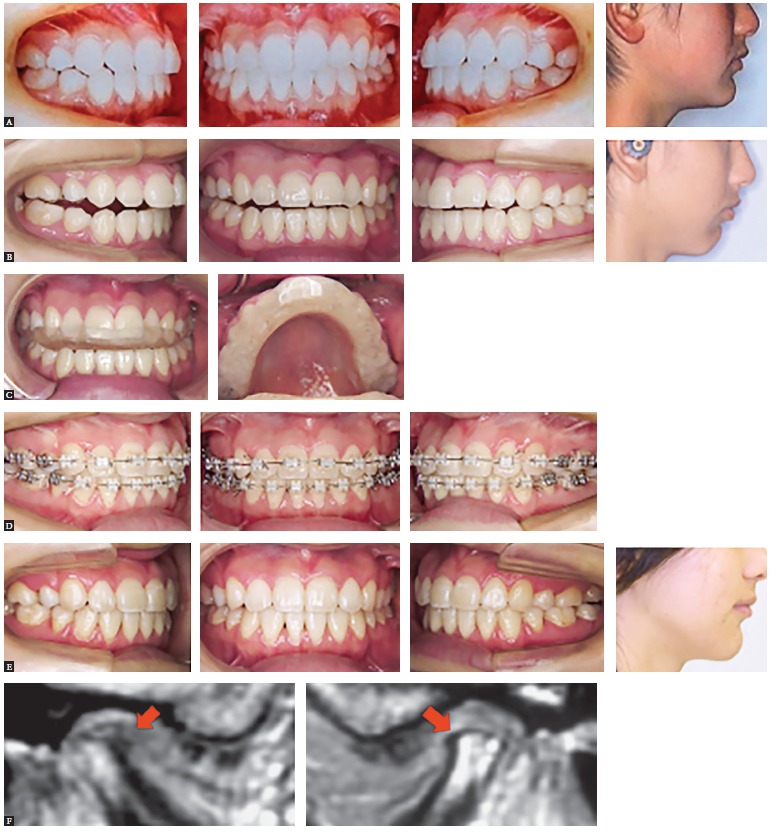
A treatment case with open bite accompanied by osteoarthritis of the temporomandibular joint. A) Initiation of retention at a previous hospital. B) Initial arrival at our hospital. C) Initiation of splint therapy. D) Orthodontic treatment with multi-bracket appliances. E) Removal of all appliances. F) MRI indicated TMJ-osteoarthritis on both sides at initial arrival (Source: Tanimoto^2^, 2011).
